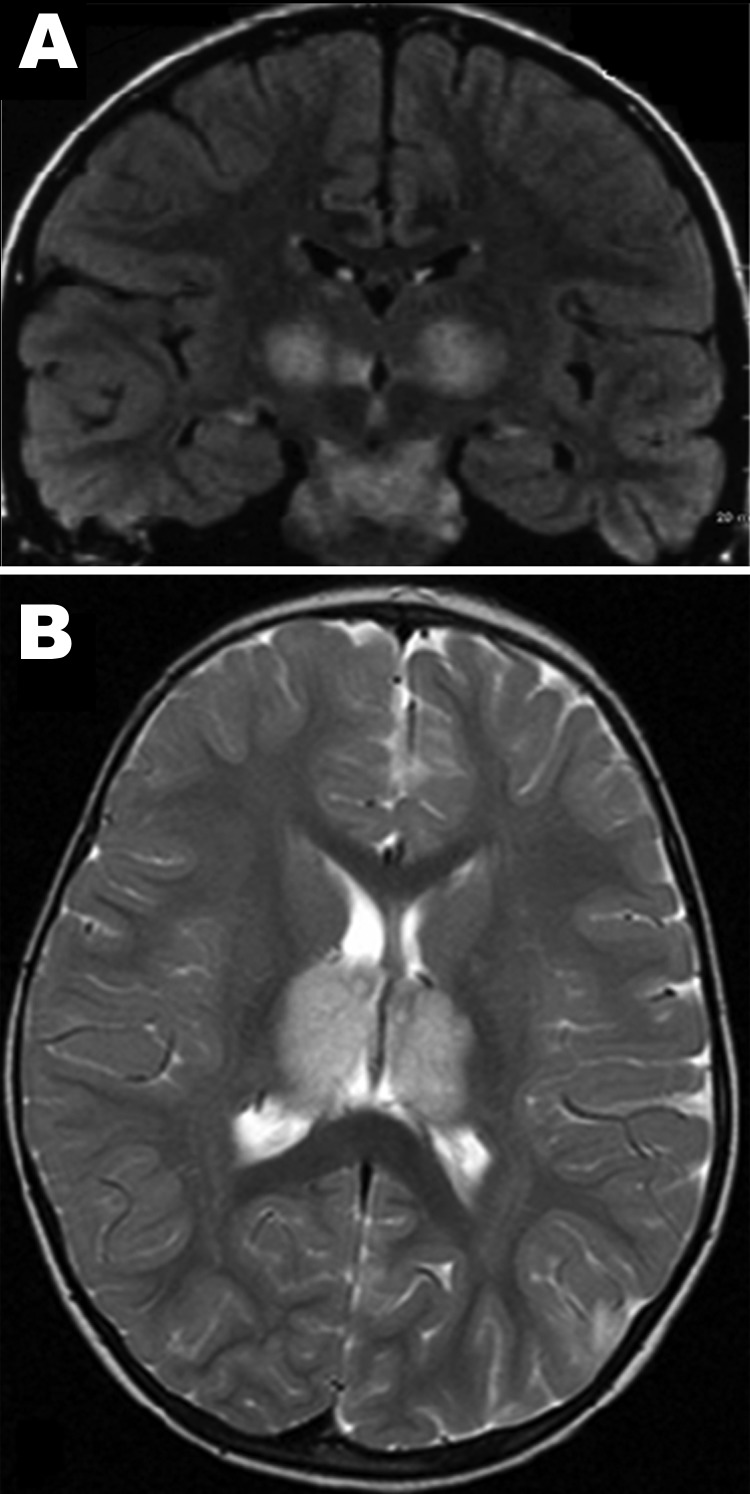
A) Magnetic resonance image of brain corona of patient 1, a 6-year-old boy with acute necrotizing encephalopathy (ANE). B). Axial-weighted images of brain thalami of patient 2, a 22-month-old girl with ANE, the cousin of patient 1.
